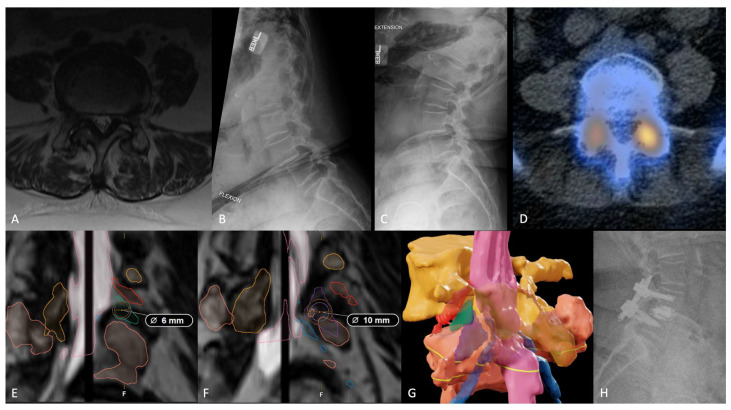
Axial T2-weighted MRI slice at L4–L5 (A) demonstrating severe bilateral lateral recess stenosis. Flexion (B) and extension (C) plain films demonstrating a grade 1 spondylolisthesis at L4–L5. CT-SPECT (D) depicting increased radiotracer uptake at the bilateral L4–L5 facet joints, left greater than right. Neurosegmentation of the left trans-Kambin’s triangle corridor ((E), green triangle), offering a maximum cannula diameter of 6 mm. Segmentation of the left transfacet corridor ((F), purple triangle), offering a maximum cannula diameter of 10 mm. Three-dimensional neurosegmentation of L4–L5 (G) showing the thecal sac (pink), left Kambin’s triangle (green triangle), left transfacet corridor (purple triangle), L4 nerve root (red), and bilateral L5 nerve roots (blue). Postoperative upright lateral lumbar plain film (H) demonstrating adequate hardware placement.
